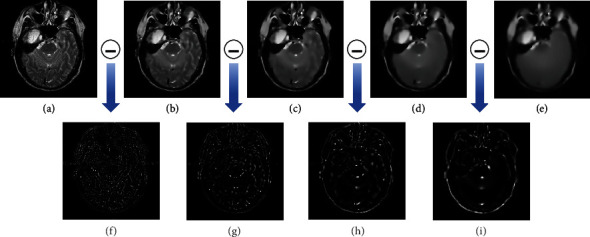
(a) MRI image. (b), (c), and (c) and (e) are the output results obtained by 4 iterations of IRGF, respectively. (f), (g), (h), and (i) are the difference between (a) and (b), (b) and (c), (c) and (d), and (d) and (e). Notice that the data of (f), (g), (h), and (i) are multiplied by 5.
